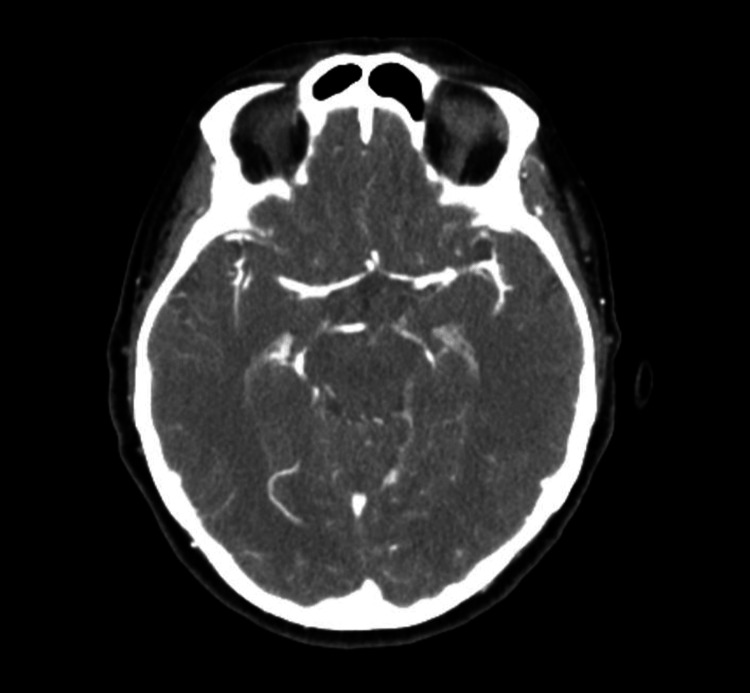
Axial CT angiography of the head demonstrating normal intracranial arterial opacification. The major anterior and posterior circulation vessels show no evidence of stenosis, occlusion, aneurysm, dissection, or other vascular abnormality. No acute intracranial hemorrhage or mass effect is identified.
